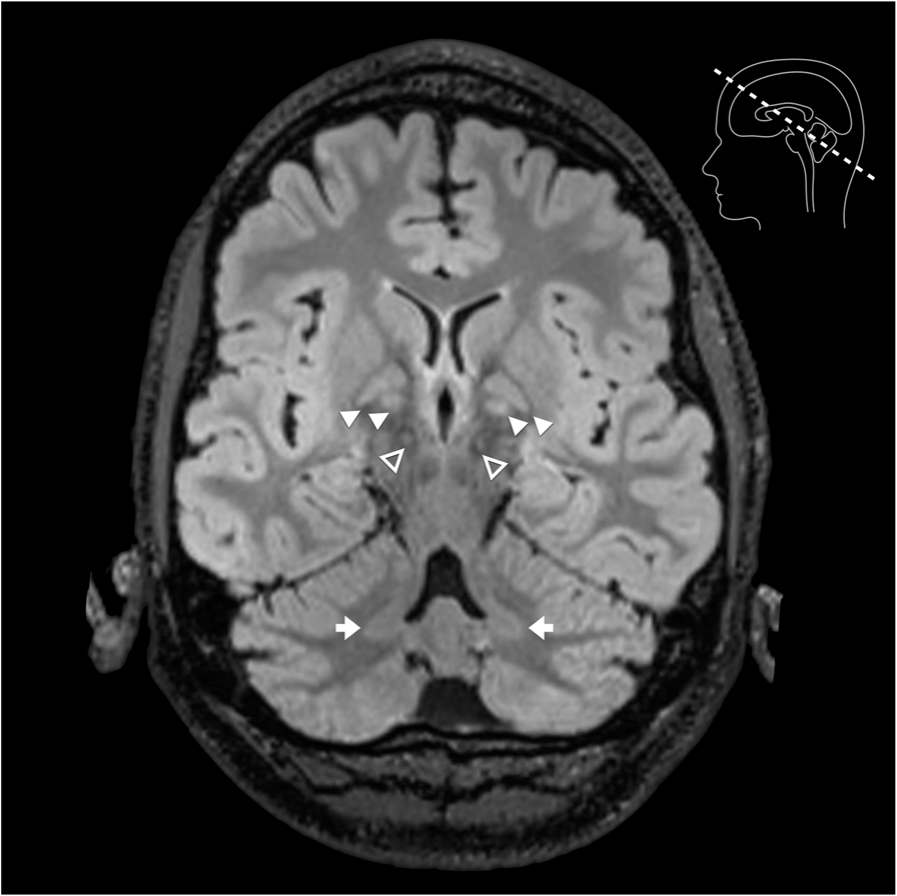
Oblique axial FLAIR image demonstrating increased T2 signal in the pars interna and externa of the globi pallidi (solid arrow heads), substantiae nigrae (open arrow heads) and dentate nuclei (arrows)
